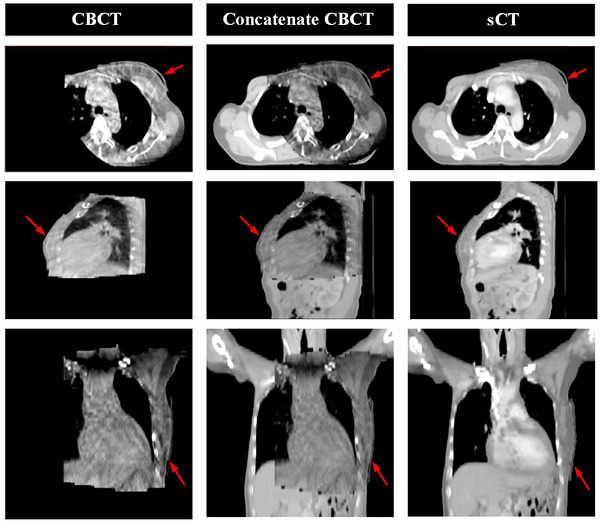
Effectiveness of converting CBCT to sCT. CBCT denotes the CBCT_1_ image acquired and registered before the first treatment fraction of patient 2 (Figure 7). Concatenated CBCT denotes the fused image of CBCT and CT images. sCT denotes the synthetic CT used for dose calculation.
